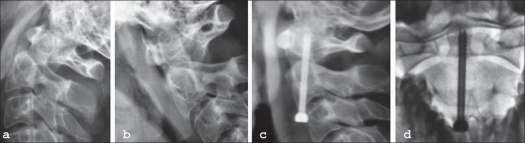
Lateral x-ray upper cervical spine in extension (a) in flexion (b) shows displaced type 2 odontoid fracture. Lateral (c) and AP x-ray (d) of the same patients after anterior odontoid screw fixation
